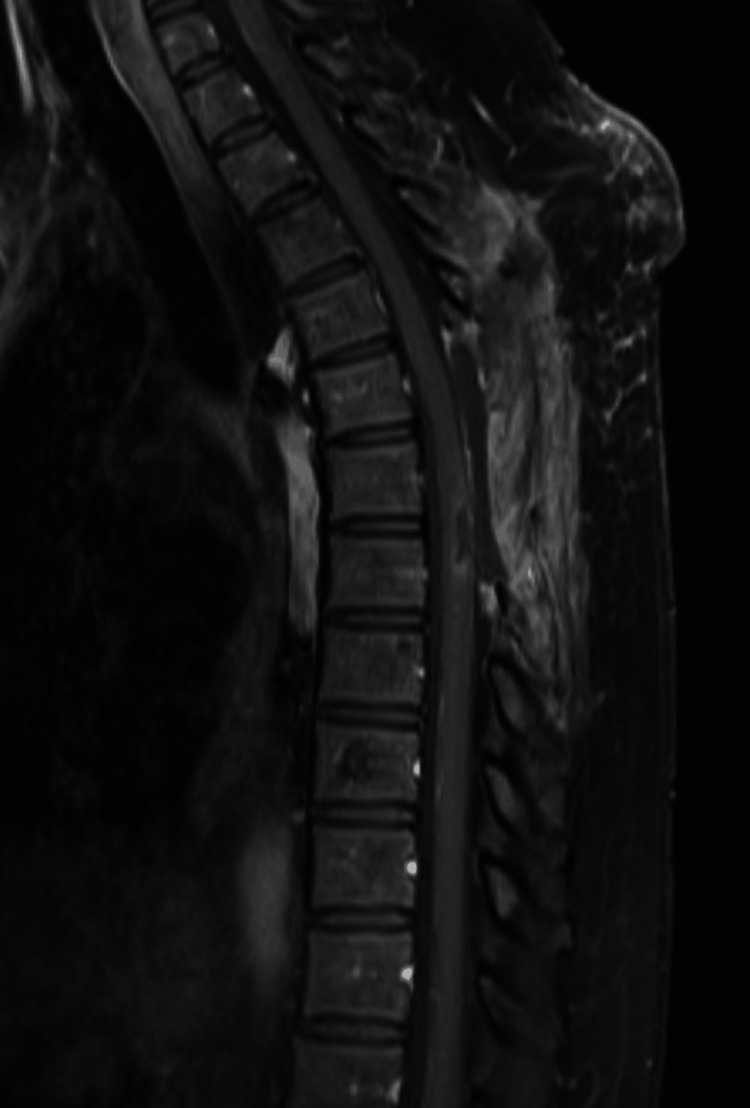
Three-month postoperative sagittal MRI negative for residual enhancement.
